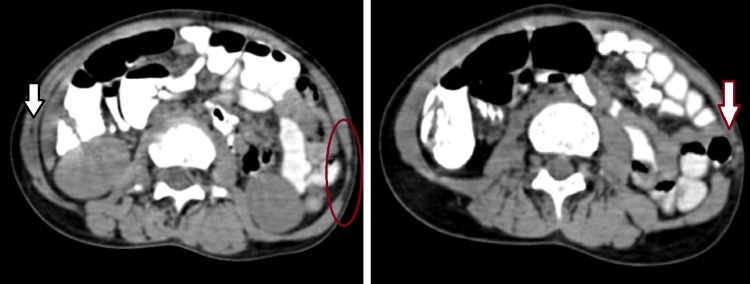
CT abdomen axial sections showing hypoplastic, thinned-out abdominal wall muscles (red circle) in the left lumbar region with herniation of bowel loops through the defect (red arrow) as compared to the normal musculature on the right side (black arrow) CT - computed tomography
